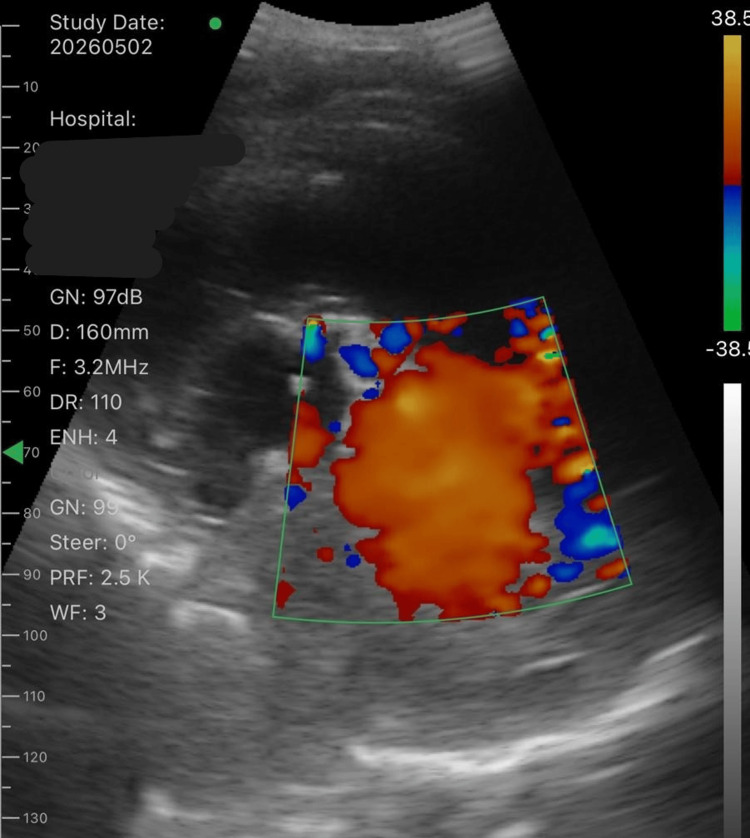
Doppler ultrasound revealing turbulent flow within a cystic structure posterior to the third ventricle, consistent with a vascular malformation.
